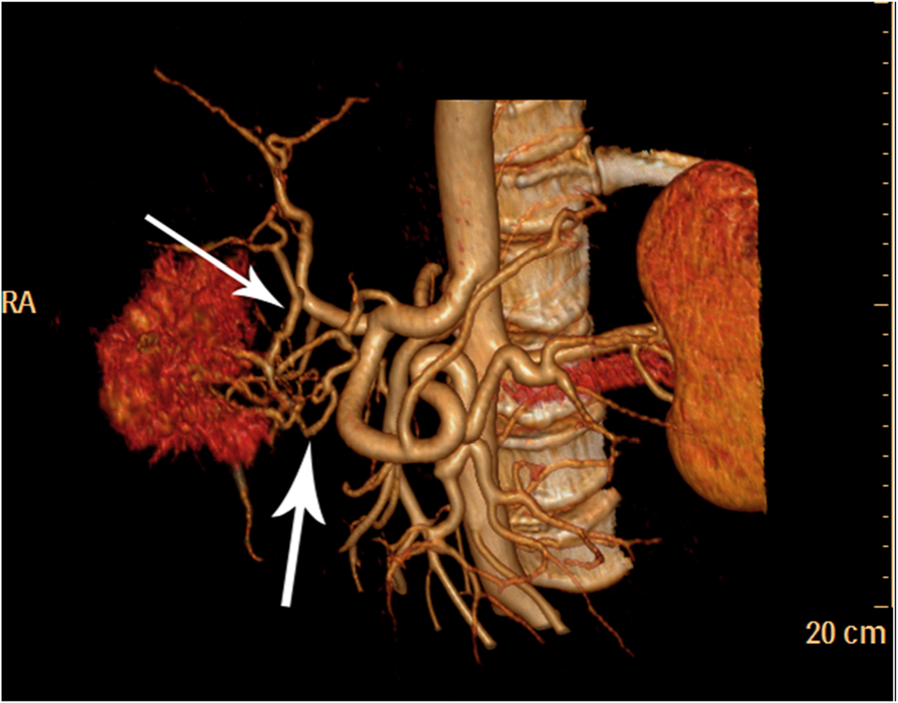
A computed tomography angiography scan showed the anterior superior pancreaticoduodenal artery (*the thin arrow*) and the left gastric artery (*thick arrow*) branches extended to the tumor center
